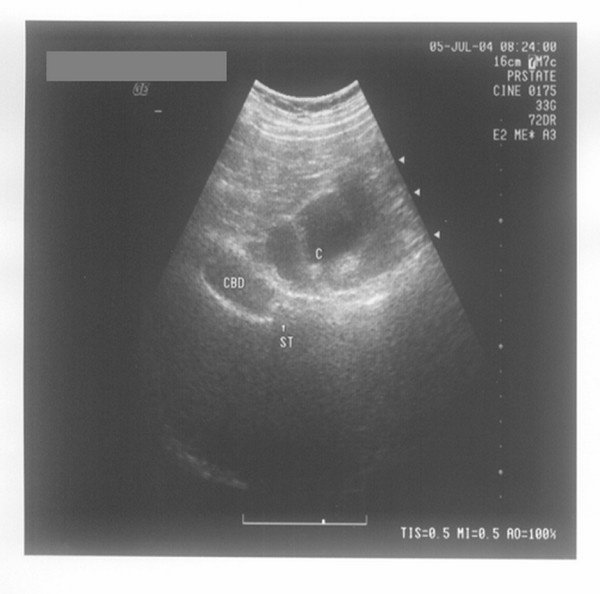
Extrahepatic bile duct calculi. Seen under transabdominal ultrasound after normal saline retention enema, sand-like calculi (indicated by ST and the arrows) accumulated at the lower common bile duct (indicated by CBD) are visible behind the transverse colon (indicated by C).
